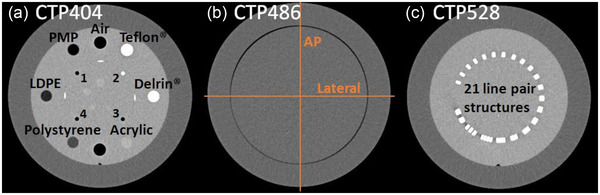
The CatPhan modules CTP404 (a), CTP486 (b), and CTP528 (c) used for physical image analysis. For uniformity assessment, computed tomography (CT)‐number profiles were drawn within the CTP486 along the indexed directions (b). The four rods used for the assessment of imaging fidelity are labeled with the numbers 1–4. Images from our archive, acquired with the conventional CT‐system SOMATOM go.Open Pro (Siemens Healthineers, Erlangen, Germany)
